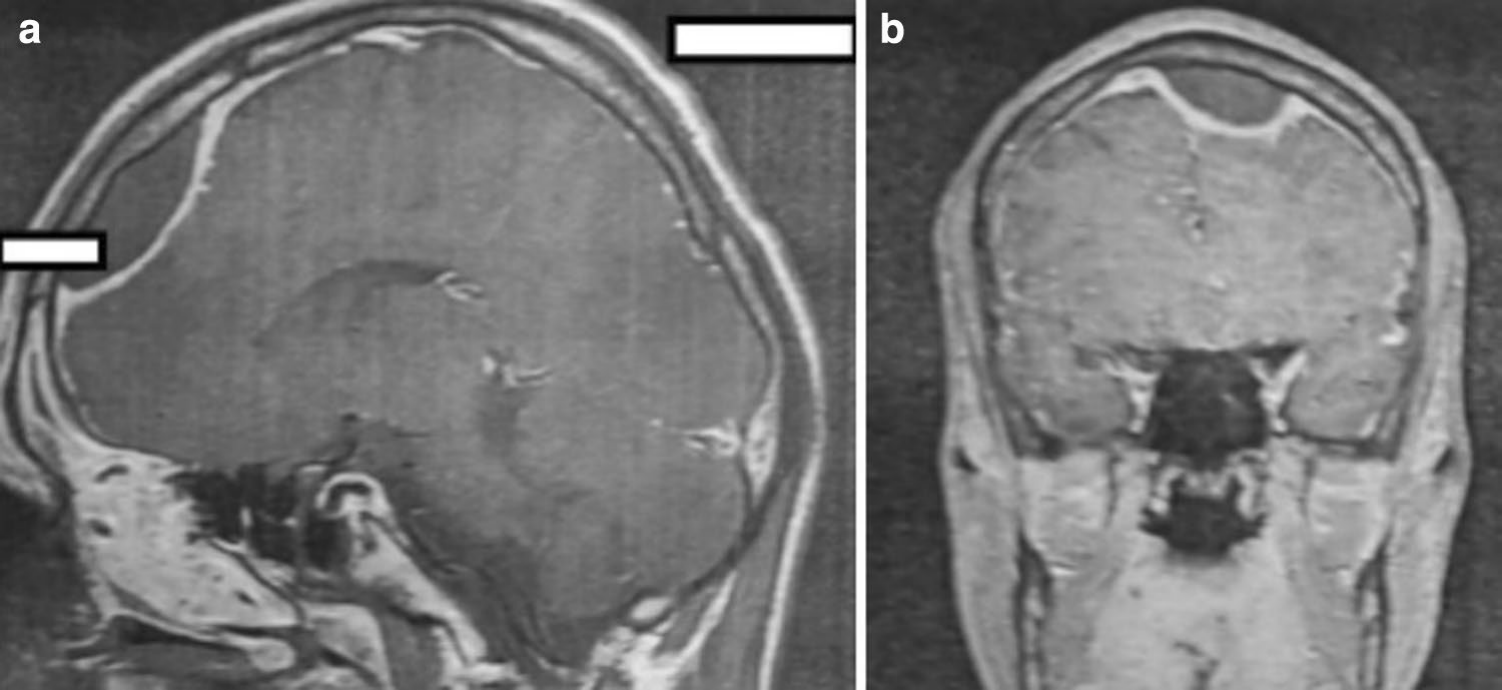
MRI of the cranium in a 20-year-old man. Abscess under the cranium near the left frontal lobe and displacement of the ventricular system to the right
